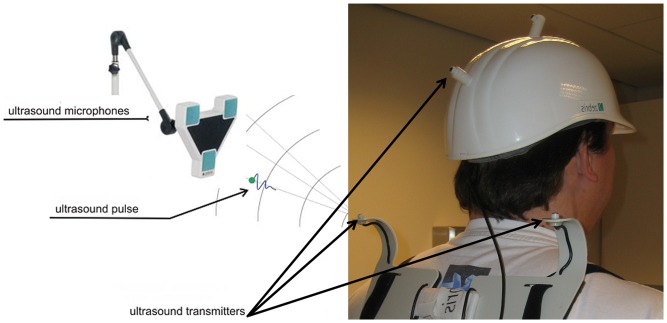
Posture analysis system. Multiple ultrasound transmitters were positioned on the body during Romberg's and Unterberger's tests; a real-time ultrasound measuring system (Zebris Medical Systems, Isny, Germany) was used to record the 3D positions of these transmitters.
